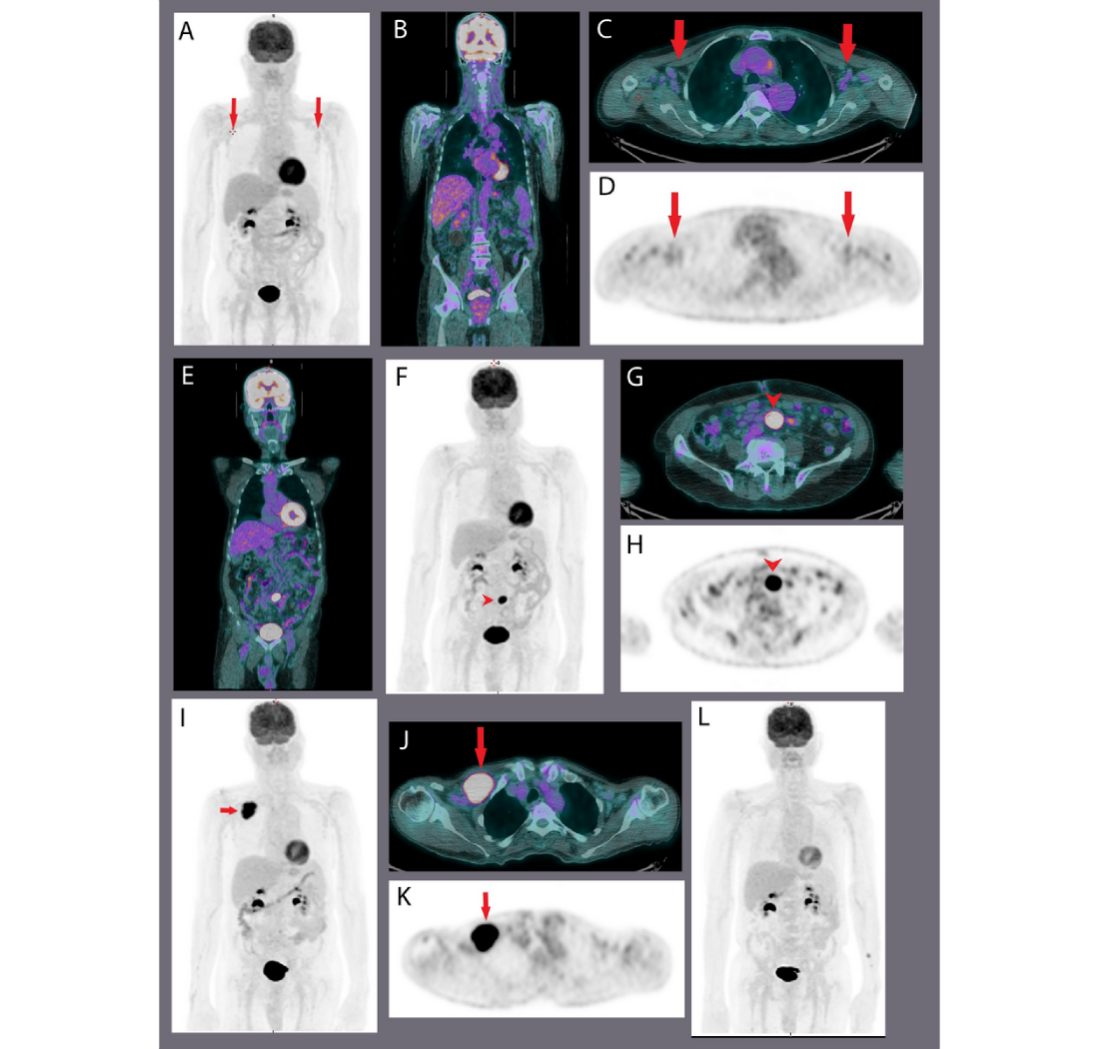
Sequential fluorodeoxyglucose–positron emission tomography/computed tomography (FDG-PET/CT) scan findings in participant number 14, a case of small lymphocytic lymphoma (SLL) and chronic lymphocytic leukemia (CLL) that transformed to diffuse large B-cell lymphoma. Initial presentation showed multiple low FDG-avid lymphadenopathy in the head and neck, mesenteric, bilateral axillary, and bilateral inguinal regions (images A-D). Solid arrows: bilateral axillary lymphadenopathy (A, maximum intensity projection [MIP]; B, coronal fused PET/CT; C, axial fused PET/CT; and D, axial PET). On follow up, interval development of new intense FDG-avid mesenteric lymph nodes (arrowhead in images E-H) and biopsy showed persistent SLL with Ki67 of 10% (E, coronal fused PET/CT; F, MIP; G, axial fused PET/CT; and H, axial PET). The patient sought a second opinion and was lost to follow-up until he reported with interval development of new intense FDG-avid large right subpectoral lymph node and interval resolution of the FDG-avid mesenteric lymph node (images I-K). Solid arrows: right subpectoral lymphadenopathy (I, MIP; J, axial fused PET/CT; and K, axial PET/CT). After R-mini-CHOP (cychlophosphamide, hydroxydaunorubicin, vincristine, and prednisone) chemotherapy, there was complete metabolic response of the previously noted intense FDG-avid subpectoral lymph nodes as well as amild FDG activity in the bilateral axillary lymph nodes (L, MIP).
